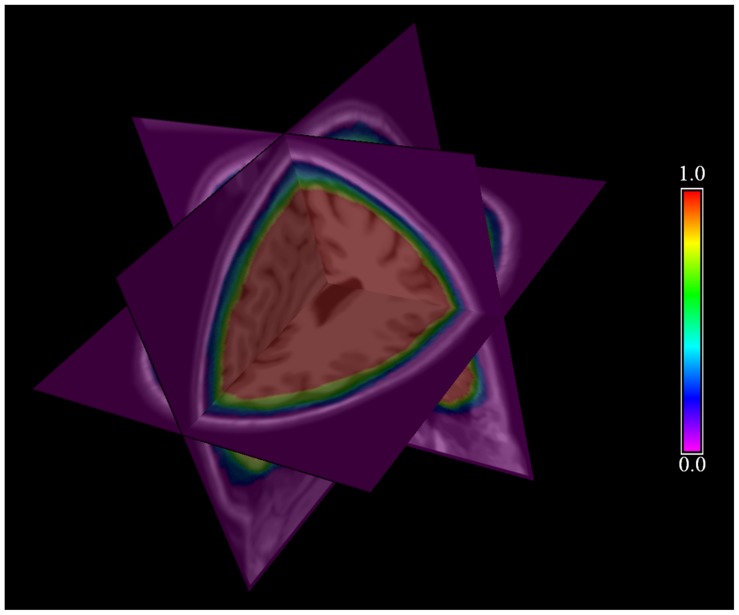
Axial, sagittal and coronal views of the brain probability map, overlaid on a brain MR image.
